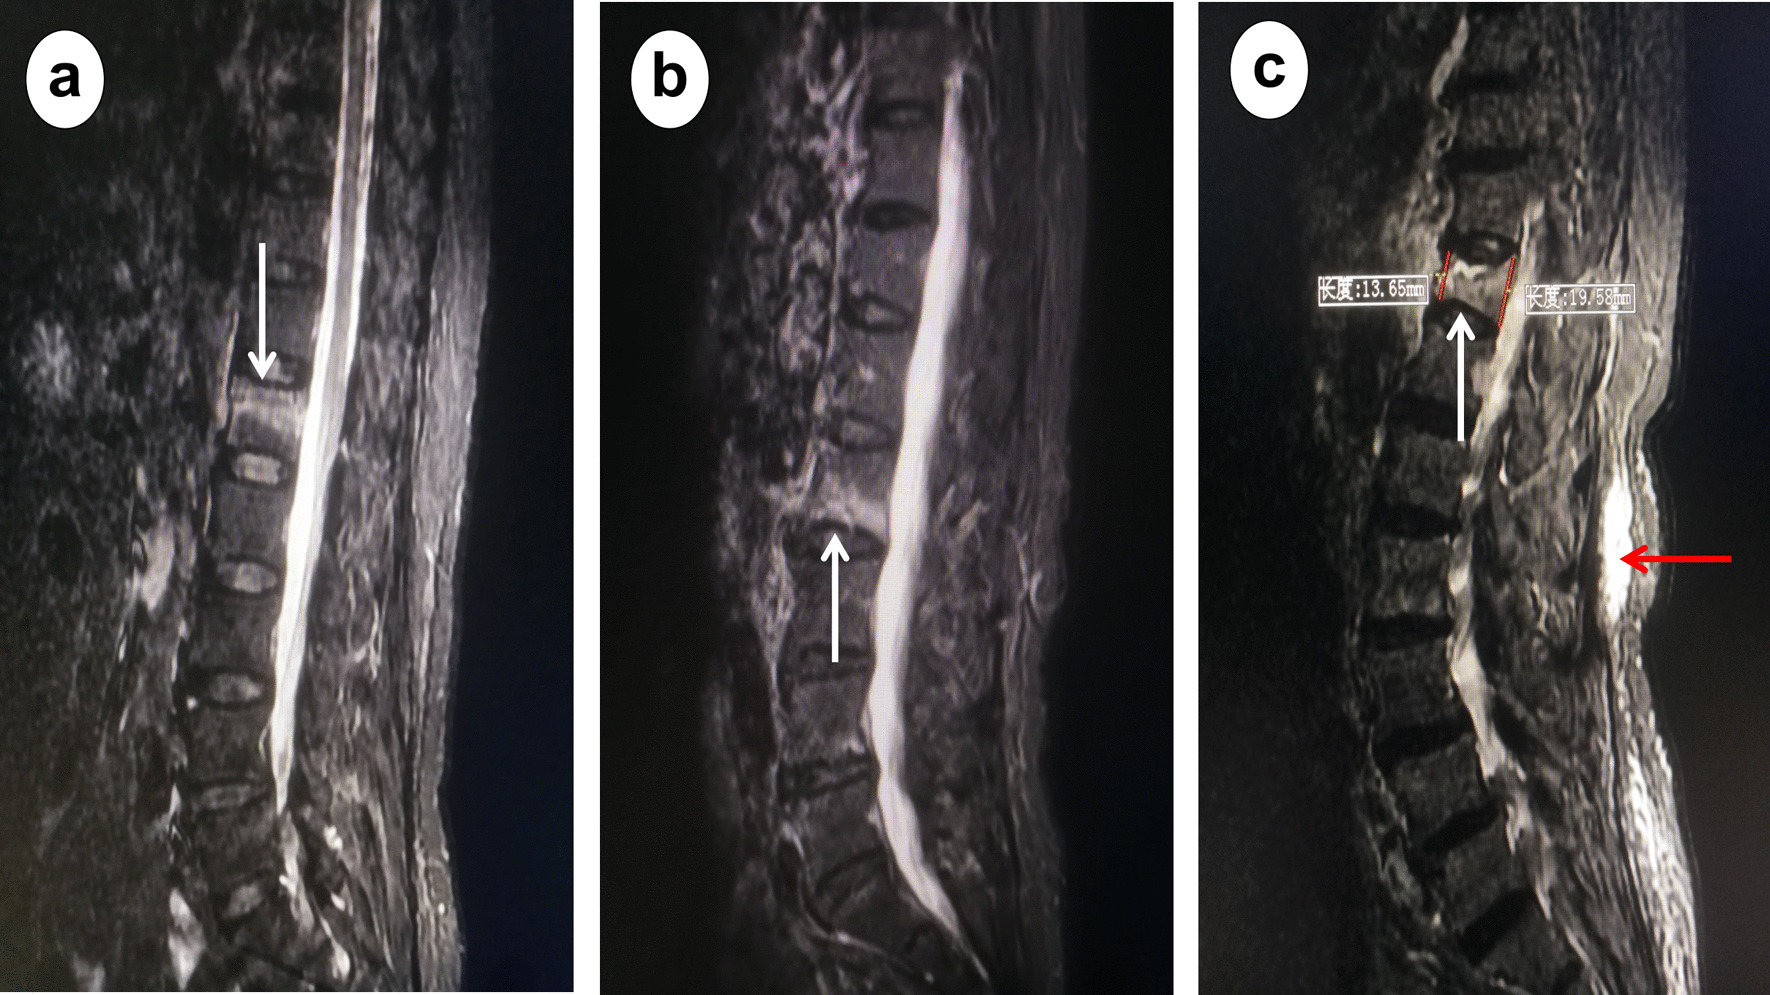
Extent of vertebral bone marrow edema and degree of vertebral compression. The sagittal T2-weighted fat suppression MRI of lumbar spine (a-c). A 72 years old female complaining back pain for 1 day after fell on ground showed vertebral bone marrow edema (white arrow) in the cranial half of L1 vertebrae (a). A 76 years old female complaining back pain for 10 days after fell on ground showed vertebral bone marrow edema in the caudal half of L2 vertebrae (b). A 67 years old female complaining back pain for 11 days after fell on ground showed vertebral bone marrow edema in both cranial and caudal half of T12 vertebrae (c). Longitudinal edema signals (red arrow) were detected along and dorsal to thoracolumbar fascia (c). The anterior and posterior height (red line) of T12 vertebrae was measured to quantify the degree of vertebral compression (c)
